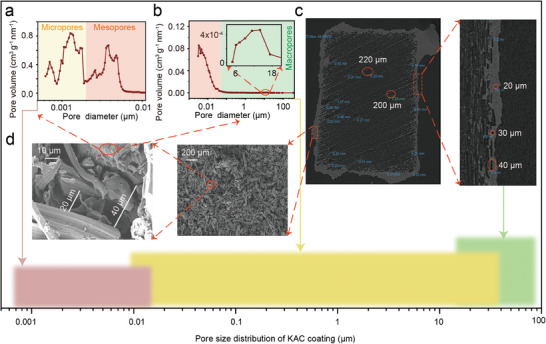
The full‐scale pore size distribution of KAC coating on native wood surface was analyzed by nitrogen adsorption method a), mercury injection methods b), X‐ray computed tomography measurement images (Slice 469/1381, c), and SEM images from a longitudinal view d).
